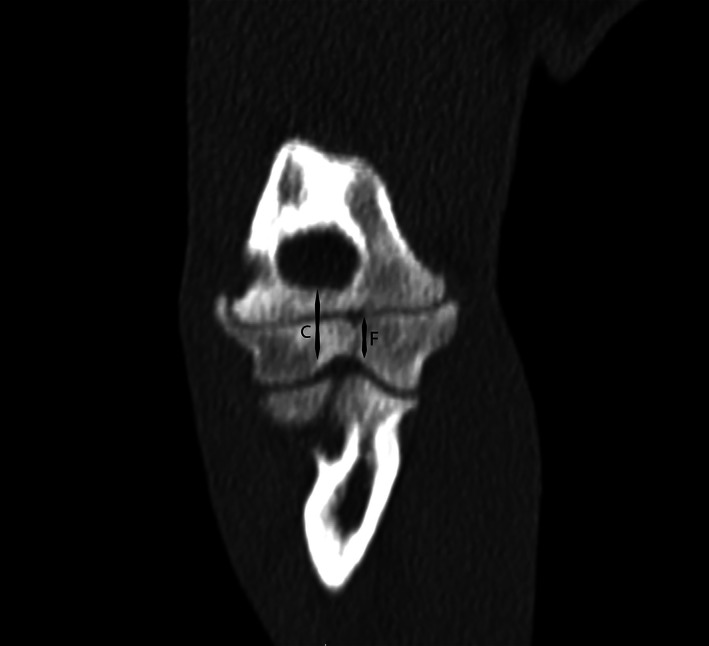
The measurements taken from the contralateral limb CT scan. This shows the measurements taken including the condylar height (C) and the fissure height (F) in order to calculate the percentage fissure length.
